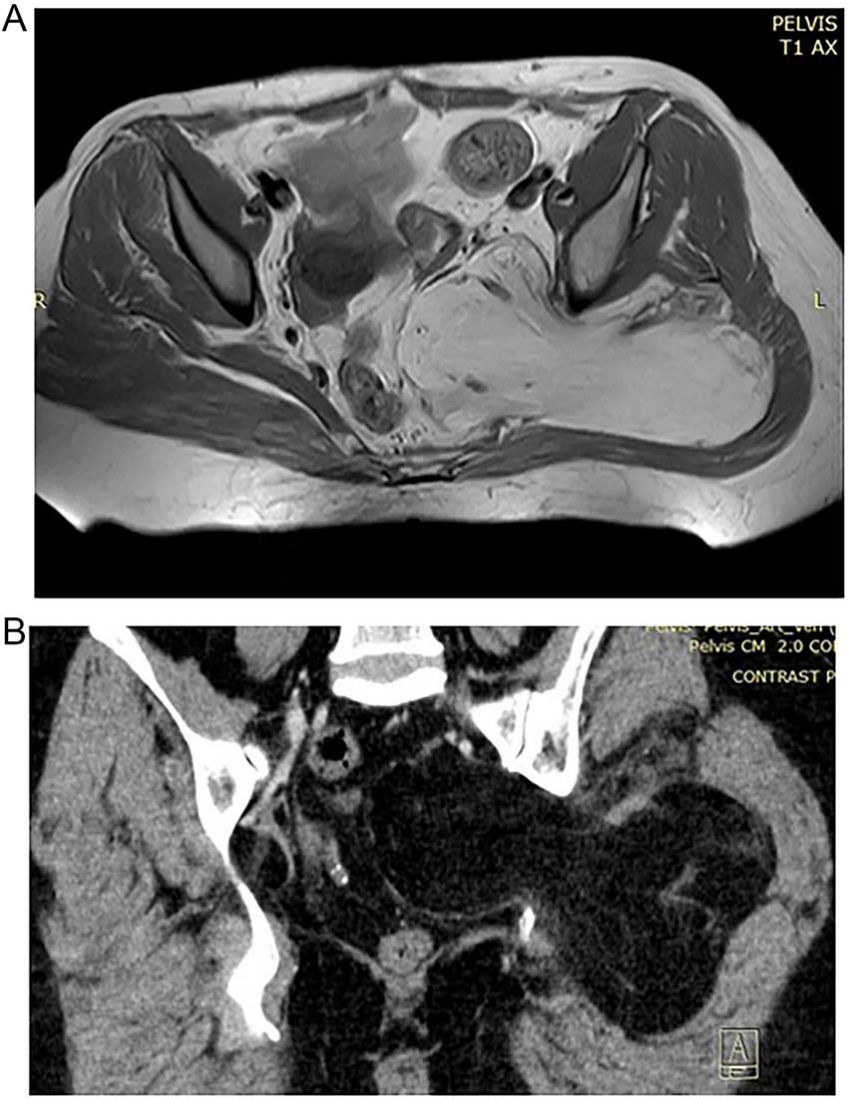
(A) Preoperative MRT image showing lipoma in the pelvic region. (B) Preoperative CT of the 57 year old patient with high signal of the lipomatous lesion.
